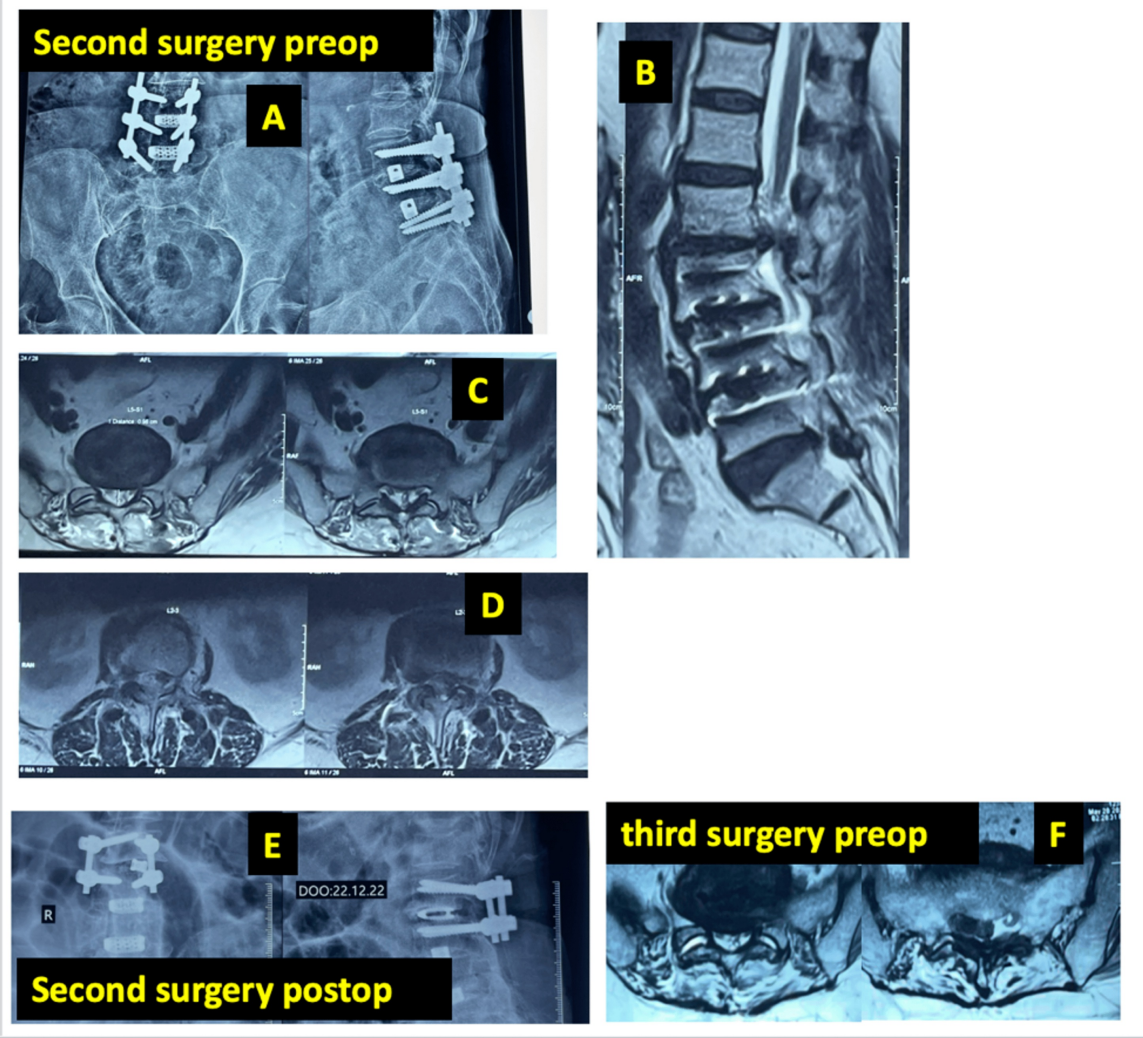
A 40-year-old male patient who underwent long fusion from L2-L5, developing a L5S1 prolapsed intervertebral disc (PID) after a two year follow-up A: Preoperative X-ray of the patient who developed adjacent segment disease (ASD) after L3-5 fusion; B, D: preoperative T2 weighted sagittal and axial MRI showing ASD at the above level during the first revision; C: axial T2 weighted MRI at the L5S1 showing healthy L5S1 at the time of first revision while addressing L23 ASD; E: postoperative X-ray after L23 fusion for the ASD; F: T2 weighted axial MRI imaging at two years after first revision showing a disc prolapse at the L5S1 level probably due to the increased stresses due to long fusion.
